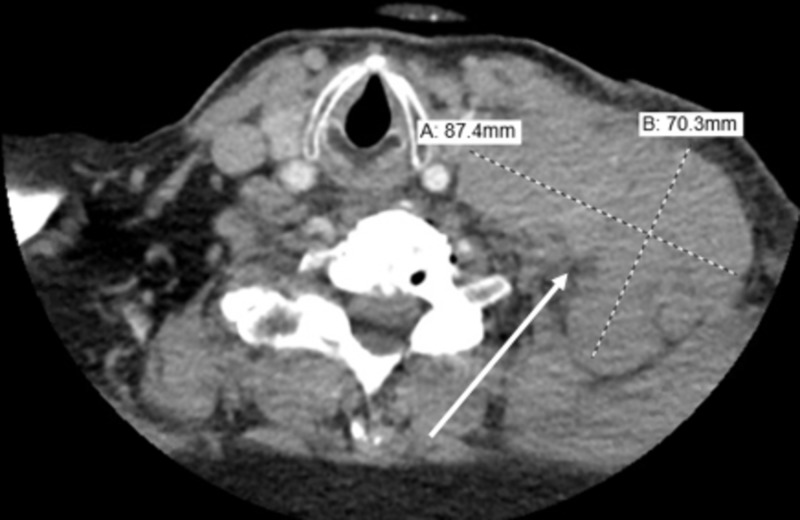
Computed tomography of the soft tissue of neck revealing an 8.7 x 6 cm soft tissue mass.
